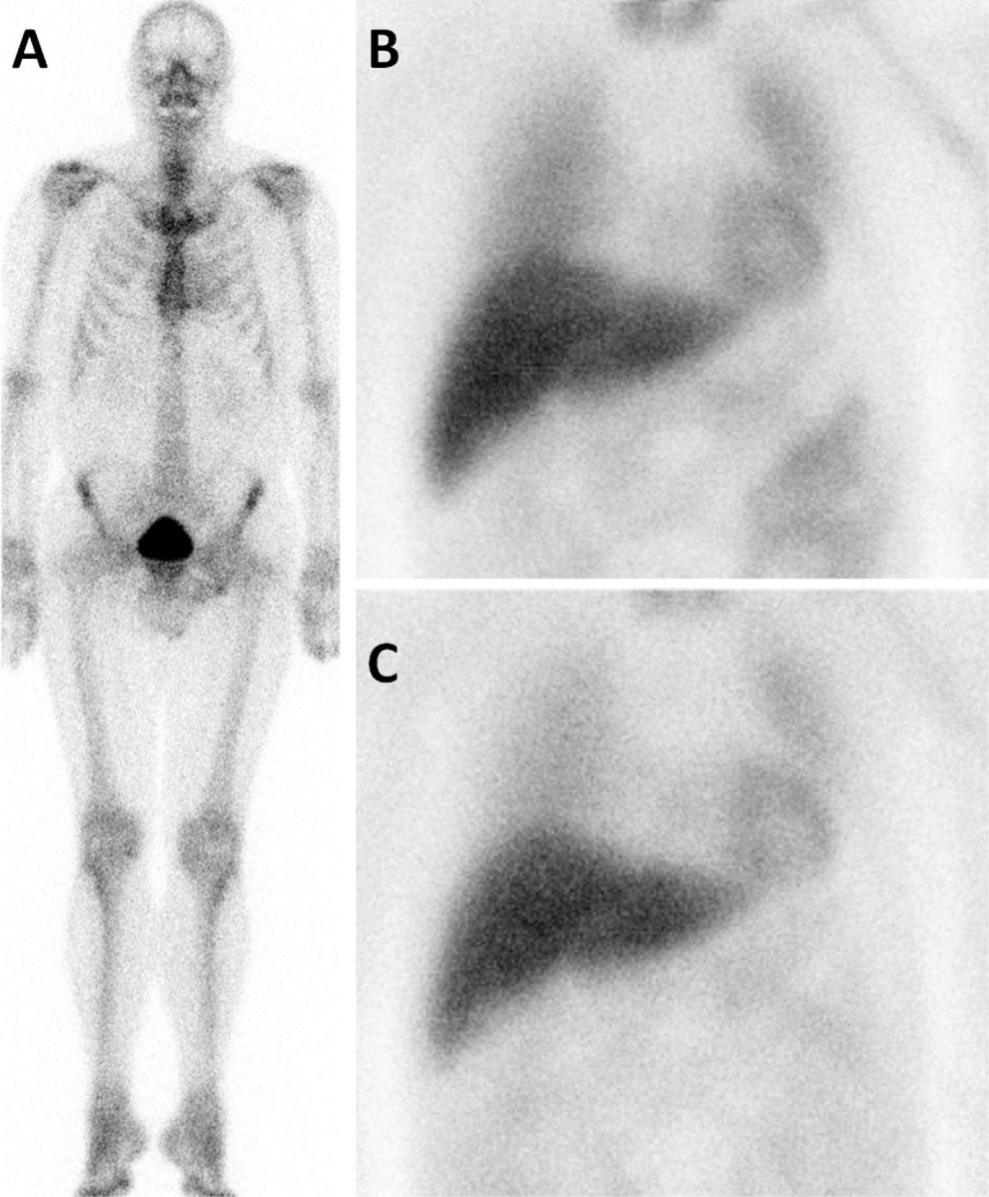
Example of a 60 year old male patients with ATTR amyloidosis based on Val50Met mutation, with slightly elevated cardiac bone tracer accumulation (A), but [I-123]-MIBG scintigraphy within normal ranges (B 15 minutes p.i., C 4 hours p.i.). Late HMR 2.2
